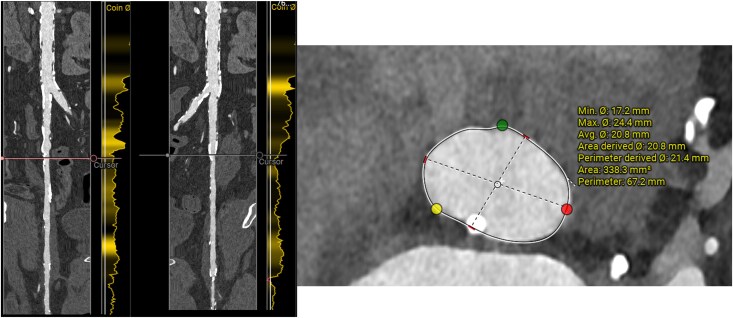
Computed tomography highlighting both the femoral anatomy, as well as the aortic annulus.
